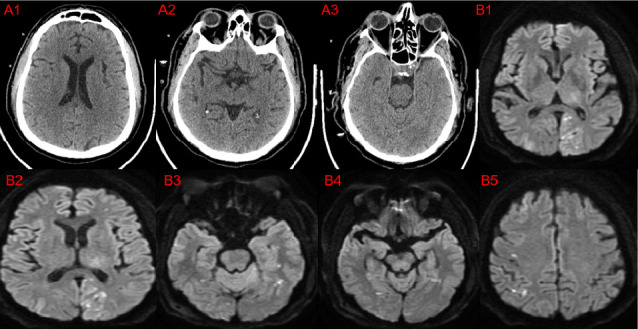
A 57-year-old man who underwent on-pump CABG and mitral valve repair with a cardiopulmonary bypass time of 221 min and aortic cross-clamping time of 191 min. Postoperative nonawakening occurred, computed tomography of the brain did not show brain vascular insult (A1–3), and CT angiography did not show large vessel occlusion. Magnetic resonance imaging of the brain (B1–5) revealed multiple patchy foci of restricted diffusion noted in the external watershed zone of both cerebral hemispheres involving bilateral frontoparietal, temporal, and occipital lobe cortices. Faint high signal areas with mild restricted diffusion are also noted in the left thalamus and both cerebellar hemispheres.
